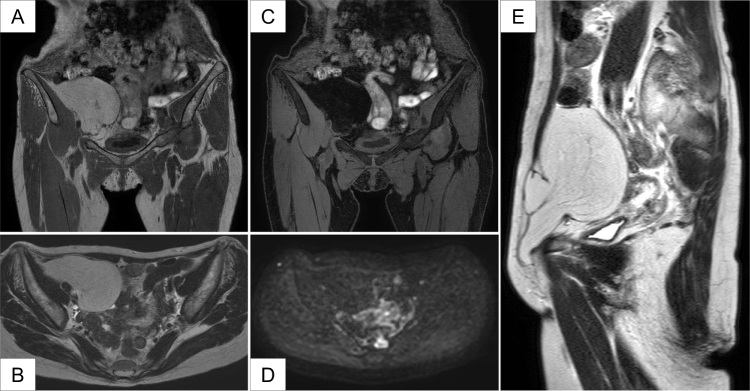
Coronal T1-weighted (A) and axial T2-weighted (B) images show a retroperitoneal mass with high signal intensity, similar to subcutaneous fat. On the coronal fat-suppressed T1-weighted image (C), the signal is uniformly low, which is consistent with a fatty lesion. The axial diffusion-weighted image (D) shows no restricted diffusion. The sagittal T2-weighted image (E) shows the tumor protruding into the right inguinal region.
